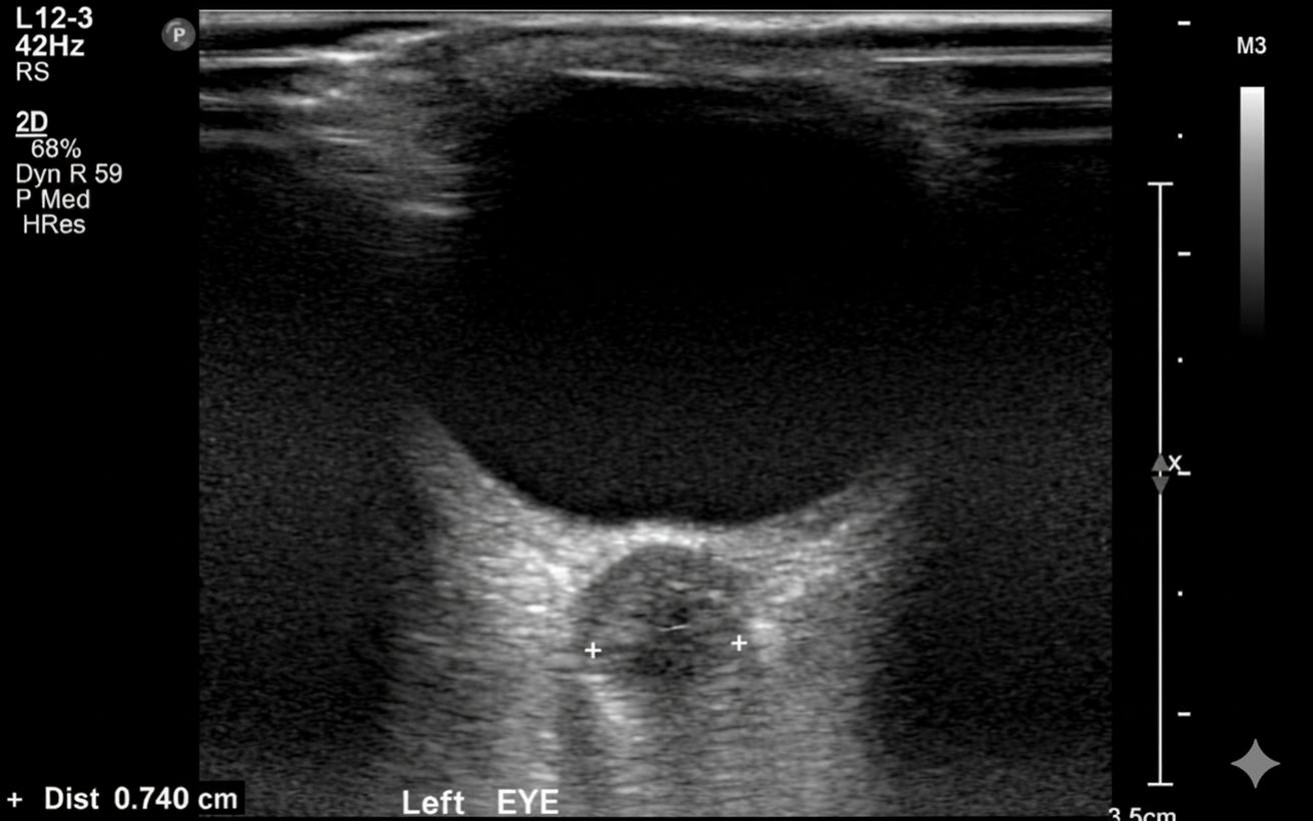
Example of blooming artefact leading to ONSD overestimation. This transverse B-mode ultrasound image of the left eye shows measurement of the ONSD at 3 mm posterior to the globe. The reported ONSD is 0.70 cm (7.0 mm). However, the hyperechoic margins of the ONS appear markedly thickened and poorly demarcated from surrounding tissue, suggesting the presence of a blooming artefact. The sheath seems disproportionately large compared to the ON itself.
